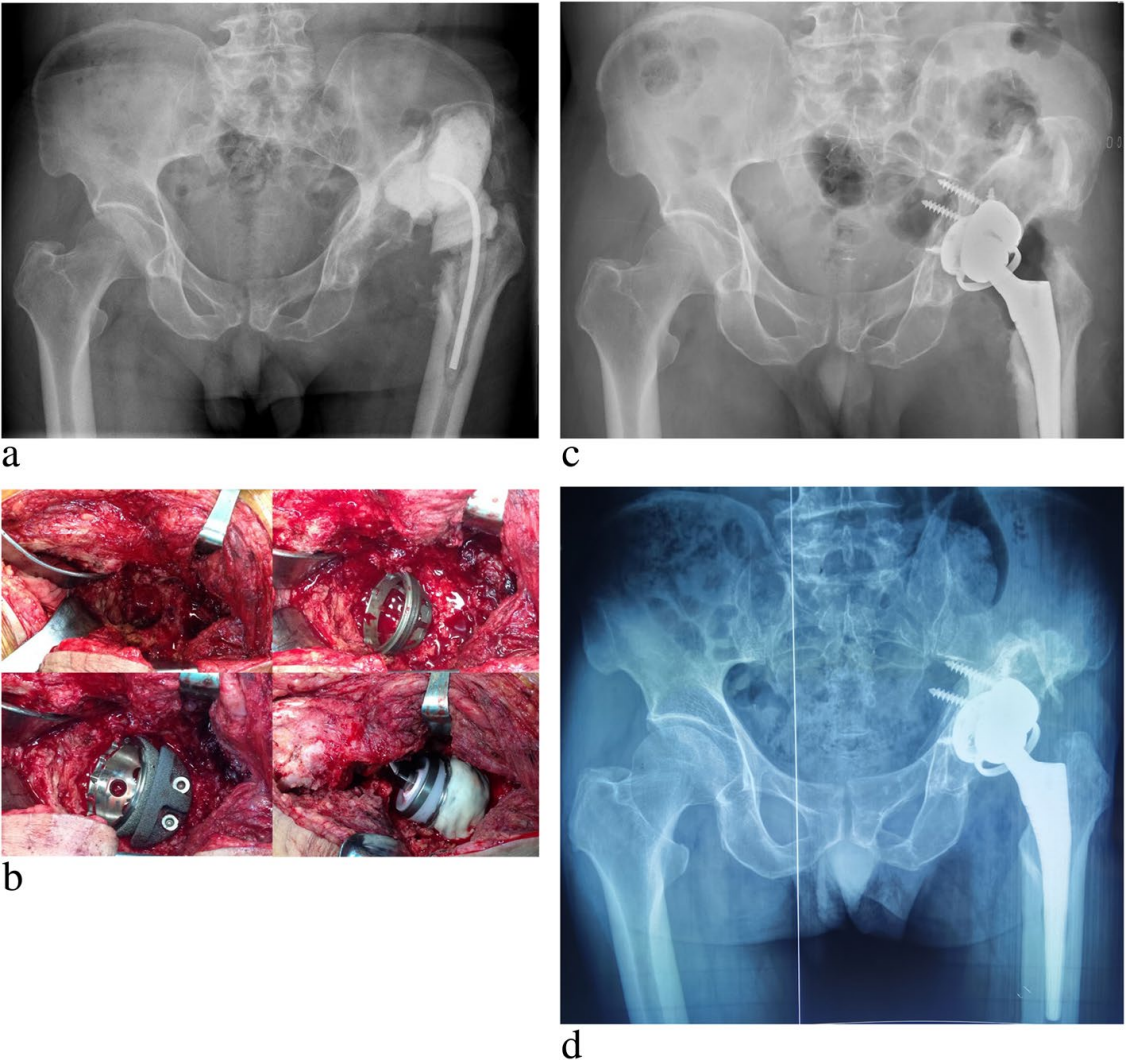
A case of a 65-year-old man (patient No. 12) with absence of gluteus medius (grade 0) and Paprosky IIIA acetabular bone defect at second-stage re-implantation, in which CAL and augment were used to reconstruct the bone defect. a Preoperative anteroposterior (AP) radiograph showing Paprosky IIIA acetabular bone defect and dislocation of the affected hip, b intraoperative finding of gluteus medius absence and a large bone defect on the top and posterior aspect of the acetabulum, c postoperative AP radiograph at Day 1, and d postoperative AP radiograph at 71 months showing acetabular radiolucent lines presenting at zone 2 and 3, and acetabular components remaining stable. HHS of the patient was 85 at the most recent follow-up
